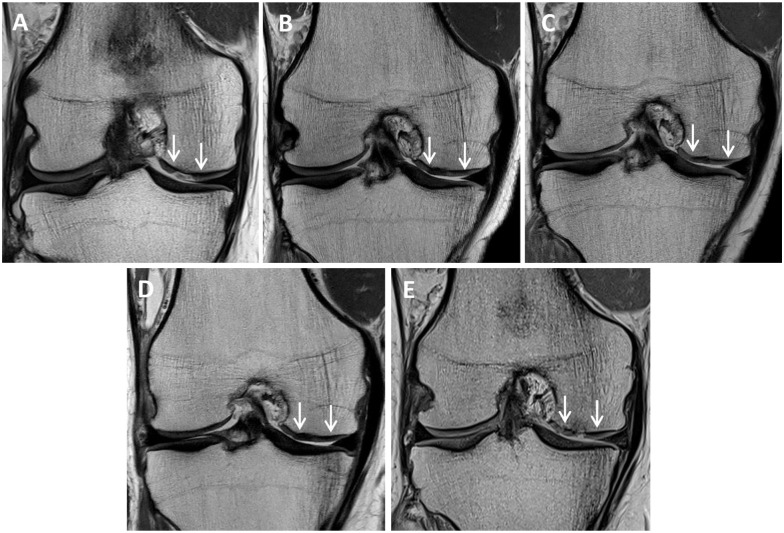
Proton density fast spin-echo magnetic resonance images of (A) a chondral defect preoperatively on the medial femoral condyle (between white arrows), as well as the subsequent matrix-induced autologous chondrocyte implantation graft at (B) 1 year, (C) 2 years, and (D) 5 years, with graft failure demonstrated at (E) 10 years.
